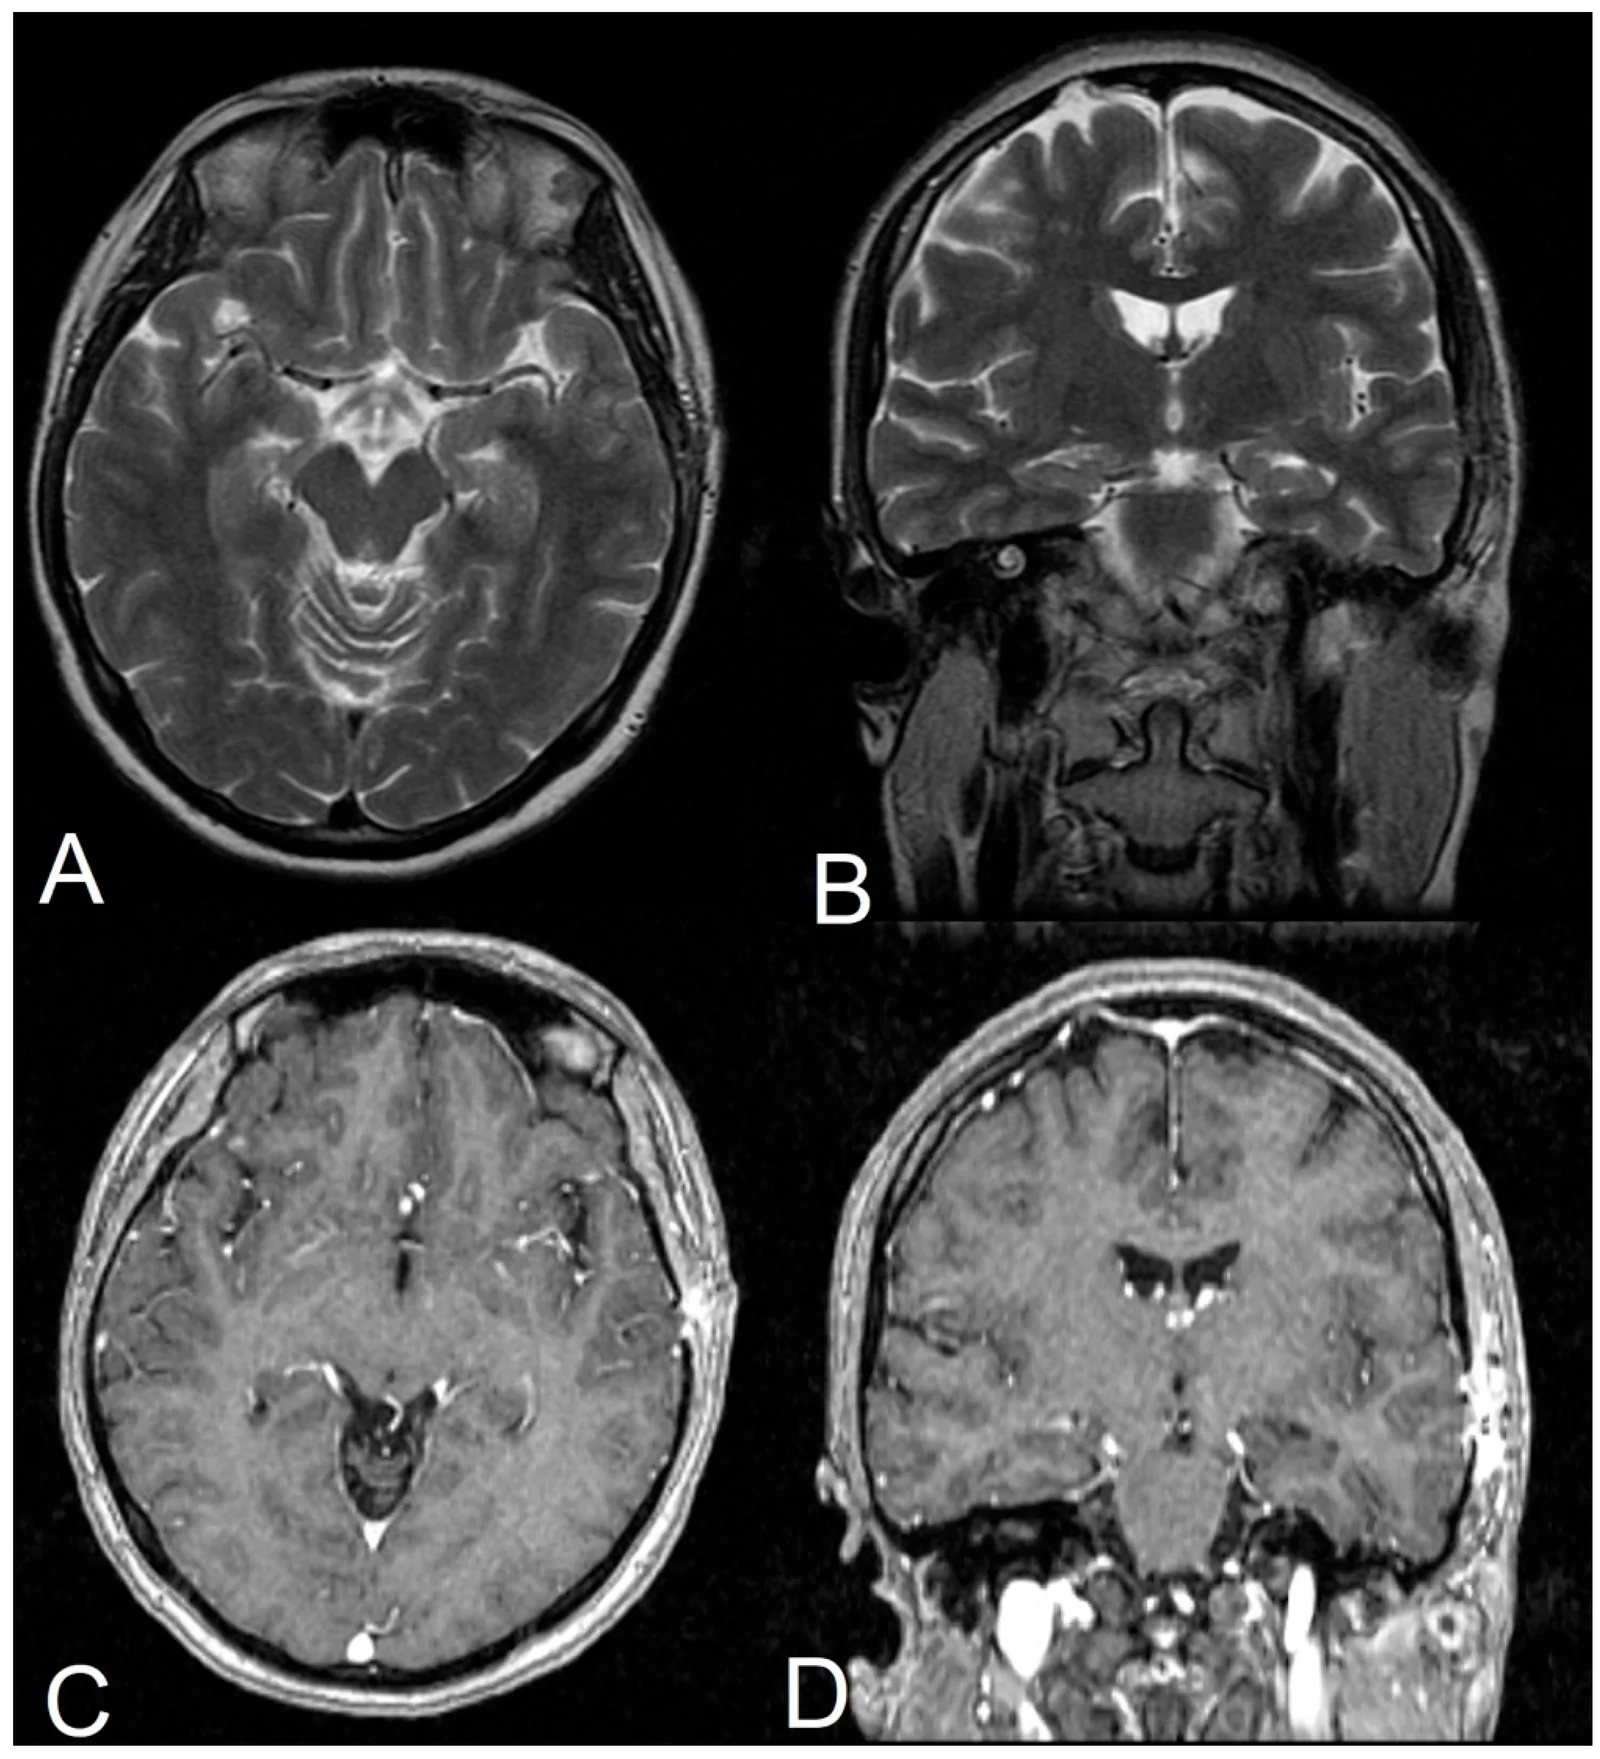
(A) Axial and (B) coronal T2-weighted images showing resolution of previously described findings. (C) Axial and (D) coronal T1-weighted post-contrast application showing minor leptomeningeal enhancement.
